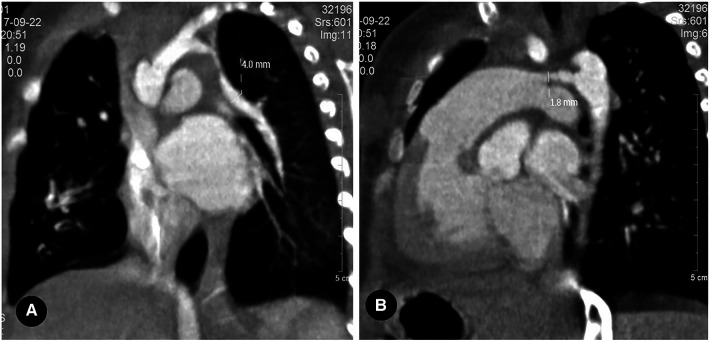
Left proximal interruption of the pulmonary artery with bilateral patent ductus arteriosus in a 4-month-old male patient. (A) a coronal view of the chest computed tomography angiography (CCTA) shows an open left ductus arteriosus connecting to the distal end of the left pulmonary artery. (B) an oblique coronal view of the CCTA shows an open right ductus arteriosus connecting the descending aorta to the proximal end of the right pulmonary artery.
